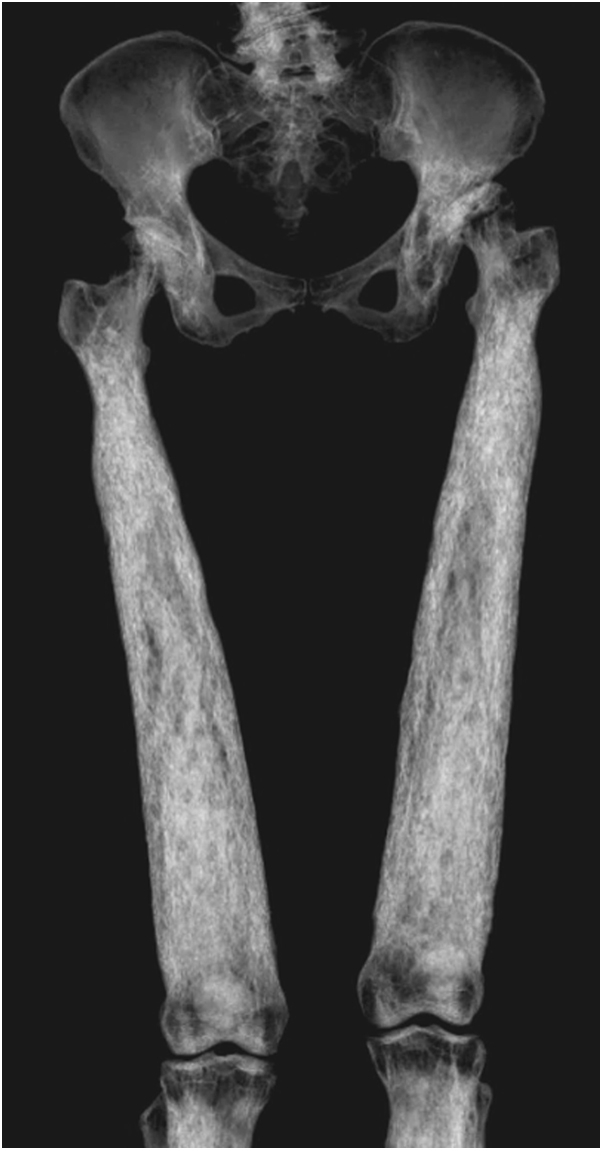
Three-dimensional reconstruction of computerized tomography (CT) scan demonstrating diffuse hyperostosis, with broadening of the femoral diaphysis.
